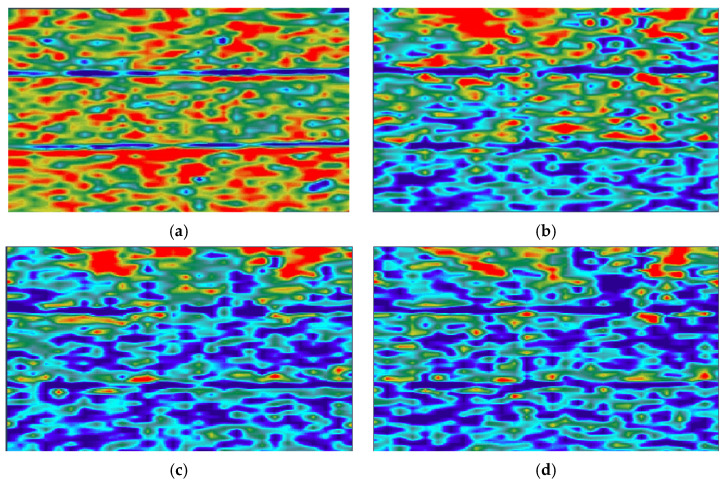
Reconstructed section of CT scan for heterogeneity modelling: (a) bound water; (b) after water flooding; (c) after SP flooding; and (d) end of flooding.
